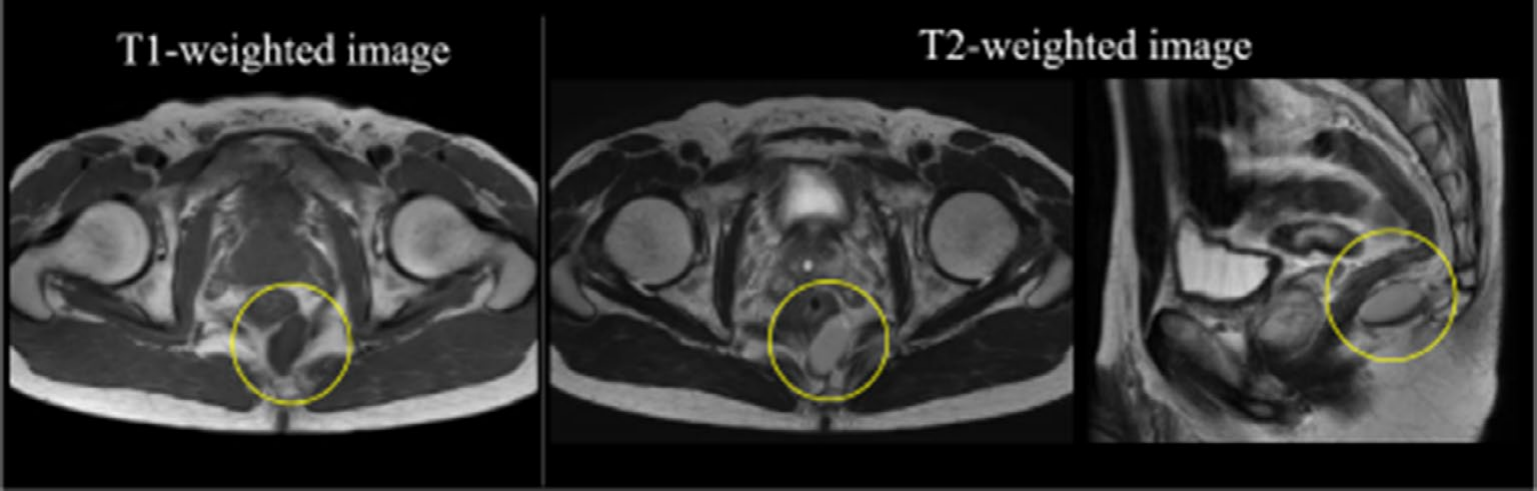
Abdominal MRI shows a cystic lesion measuring 32 × 12 × 20 mm, characterized by low signal intensity on T1‐weighted images and high signal intensity on T2‐weighted images.
